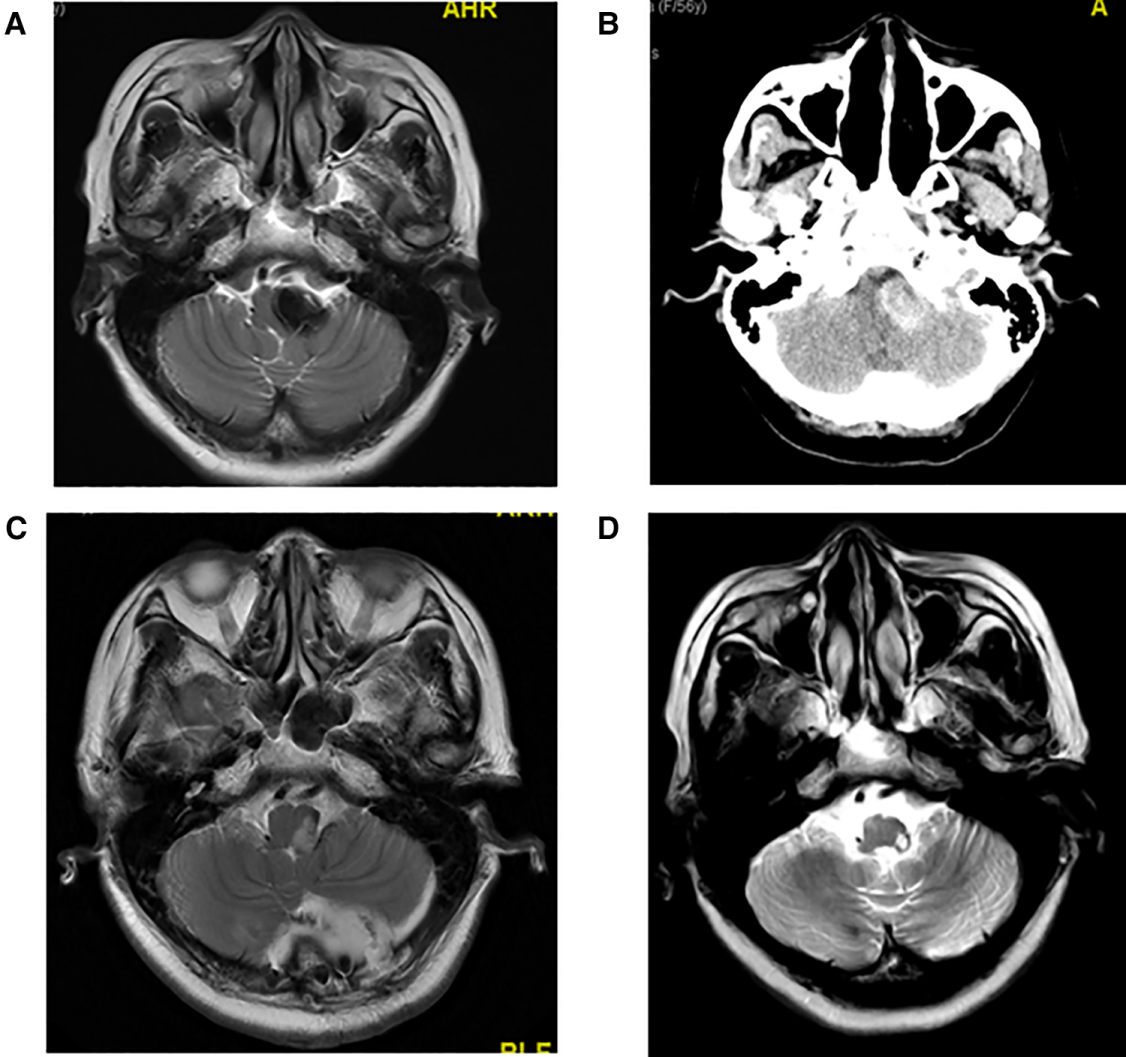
(A) Preoperative T2 MRI image of No. 13 patient. (B) Preoperative CT image of No. 13 patient with high CT signal. Hemorrhage was observed in the arachnoid cyst during craniotomy. (C) T2 MRI image of No. 13 patient at 1 week after craniotomy. (D) T2 MRI image of No. 13 patient at 1 year and 10 months after craniotomy.
